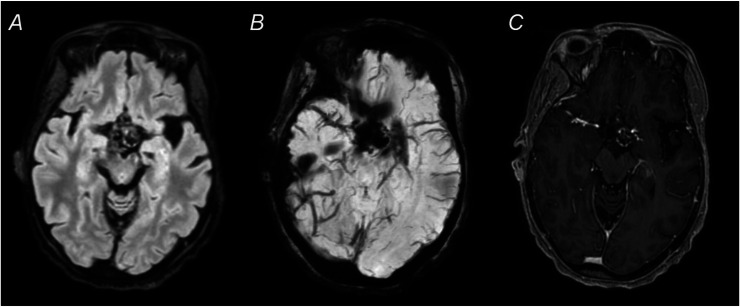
Subsequent MRI of the brain was performed with and without the administration of intravenous contrast. Axial T2-FLAIR sequence demonstrates a sellar and suprasellar mass with internal foci of low signal (A), corresponding with an area of susceptibility artifact on susceptibility-weighted imaging (SWI) sequence (B). This was compatible with the patient’s history of a treated craniopharyngioma. Mass effect on adjacent structures was causing chronic bitemporal hemianopsia and bilateral cranial nerve III palsy. Patchy enhancement was noted in the suprasellar region on axial postcontrast T1-weighted sequence (C), however follow-up over 2 decades did not demonstrate any changes suspicious for recurrent or residual tumor.
